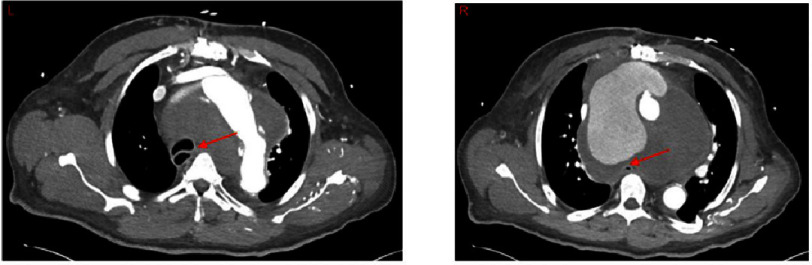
Axial Images of CT scan of a patient on VV ECMO with an aortic pseudoaneurysm at the distal suture line of a prior aortic arch replacement. Left: Aortic arch with large pseudoaneurysm and compression and distortion of the trachea (arrow) to the right side. Right: Aortic extravasation of contrast into pseudoaneurysm which can be seen compressing the esophagus (arrow). Abbreviations: CT - computed tomography, CTA - computed tomography angiogram, VV ECMO - venovenous extracorporeal membrane oxygenation.
